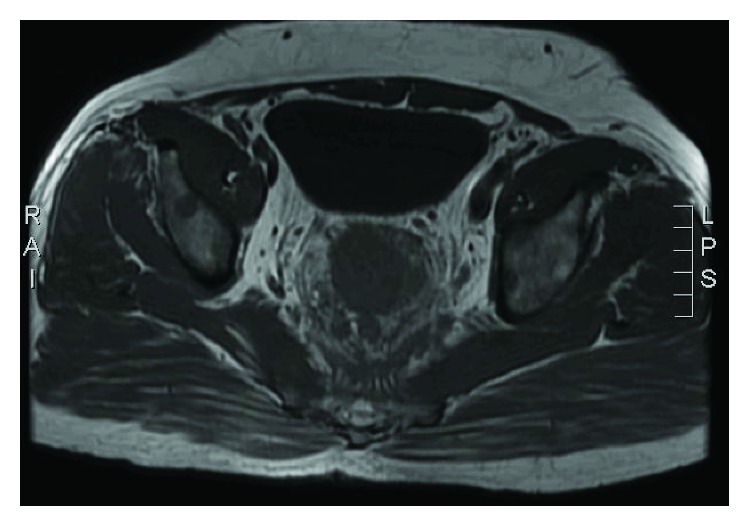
MRI showing the rectal lesion with the perirectal fat stranding.
